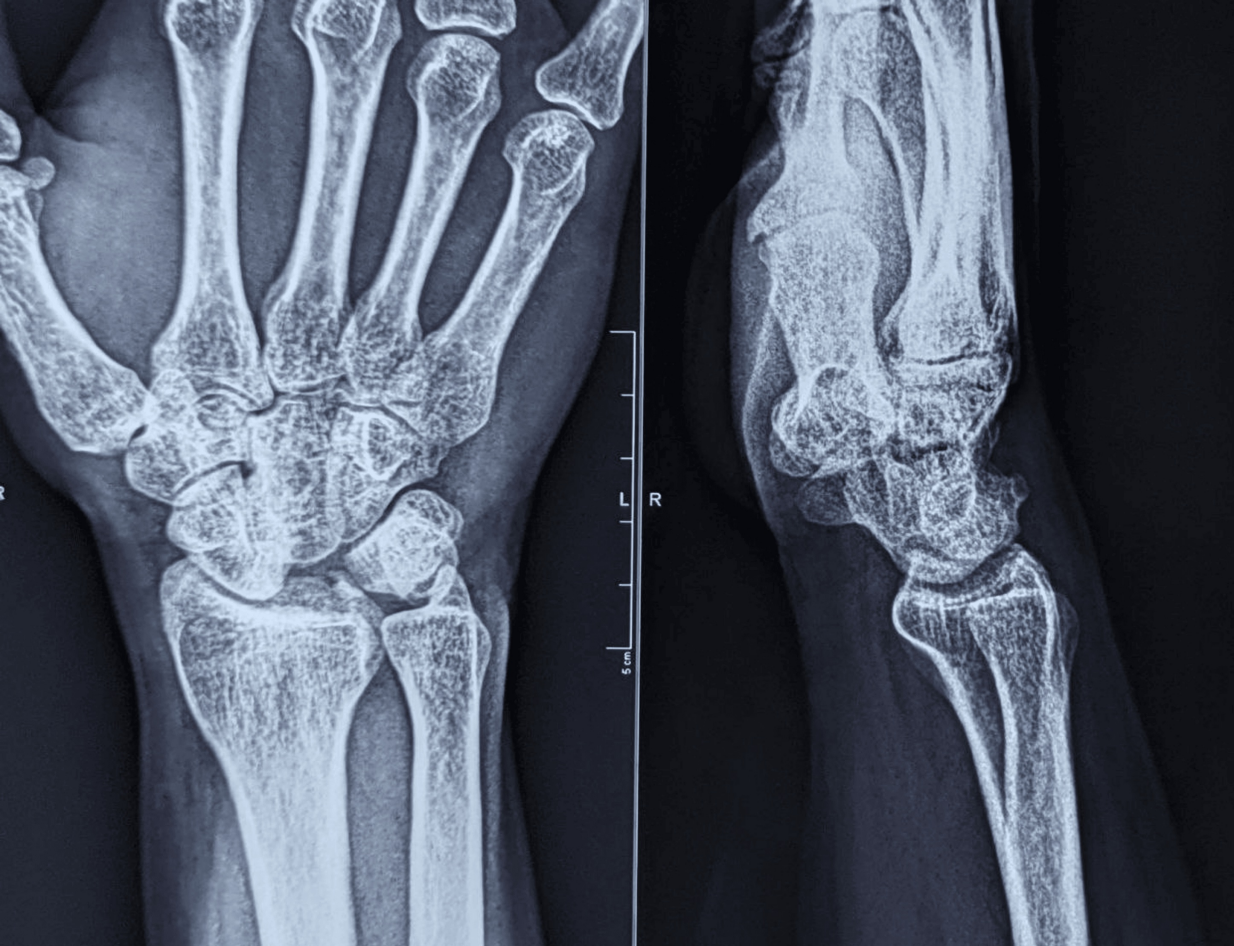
Follow-up radiographs showing solid fusion with trabeculae across the scaphocapitate articulation.
